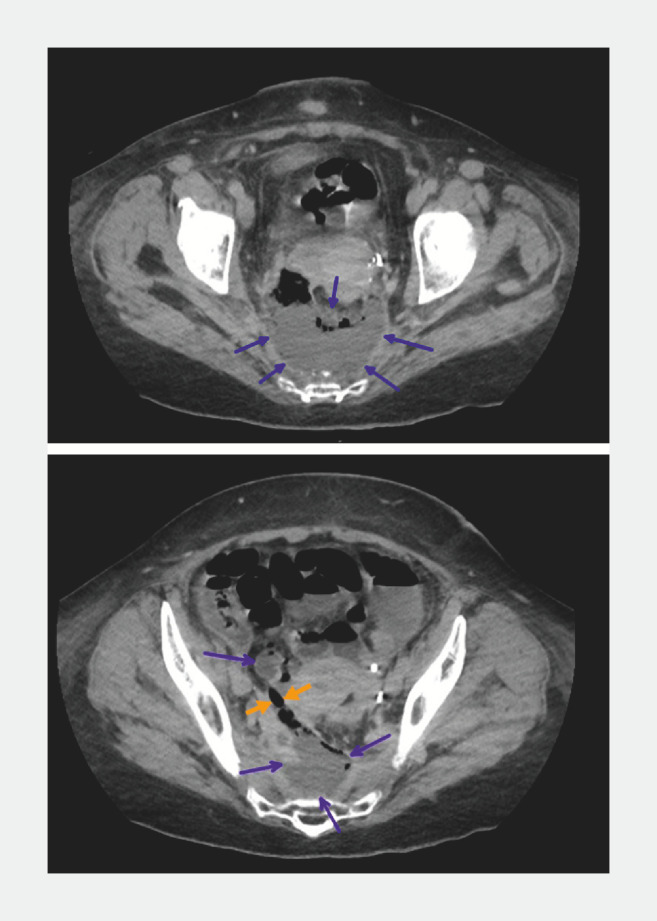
Computed tomography image before surgical drainage showing a complex pelvic collection (blue arrows) associated with a rectovaginal fistula (yellow arrows).
